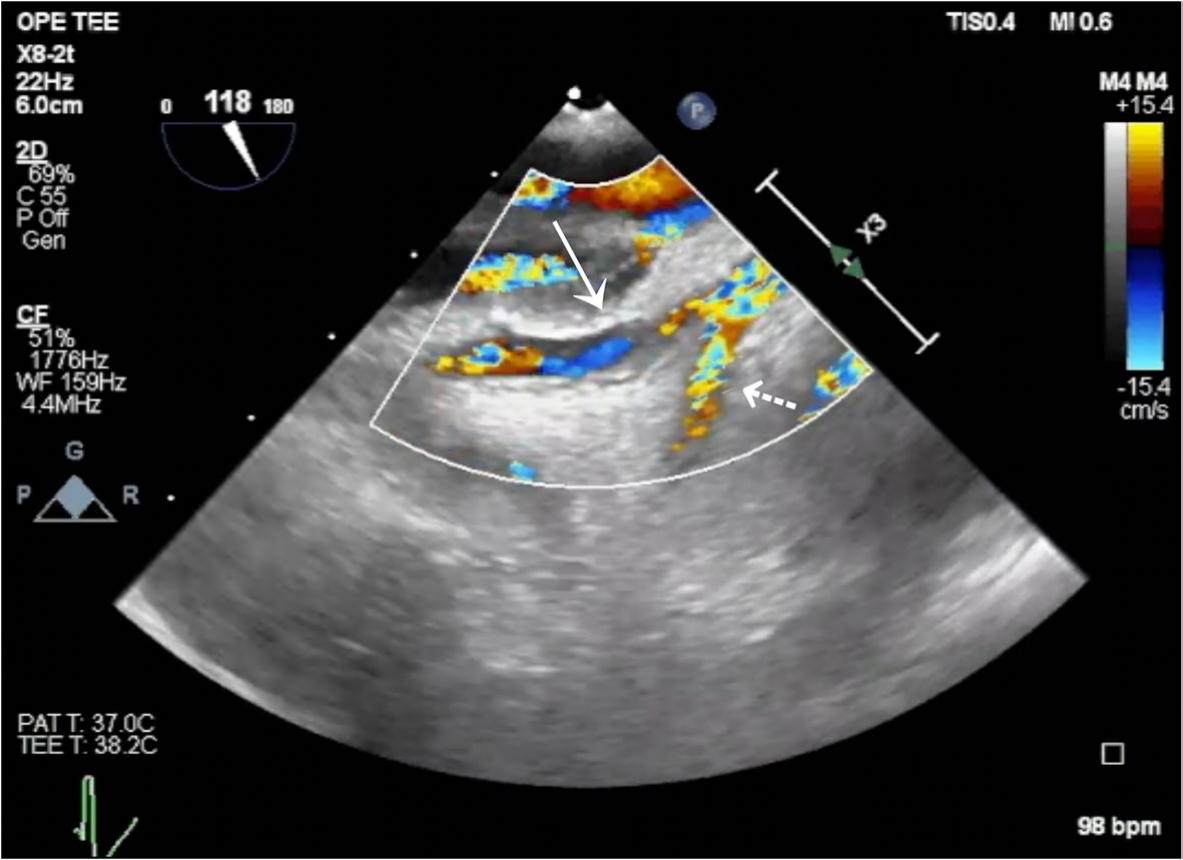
Restoration of flow in the left circumflex artery. Color Doppler image obtained using transesophageal echocardiography after removal of the implicated suture. The solid arrow indicates the left circumflex artery (LCx), and the dashed arrow indicates the left anterior descending artery (LAD)
